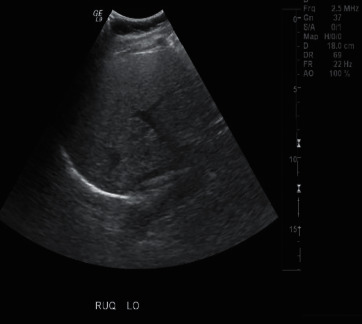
Abdominal ultrasound showing fatty liver.
